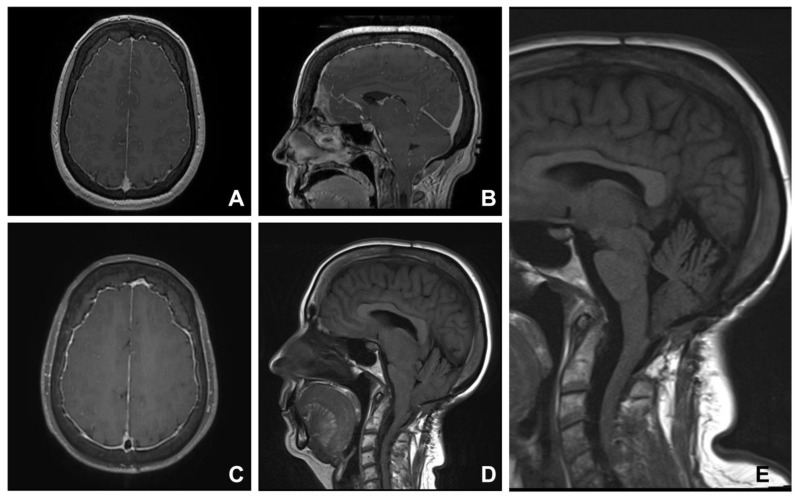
Chiari mimic: spontaneous intracranial hypotension. A 51-year-old woman was diagnosed with Chiari I malformation and syringomyelia and underwent posterior fossa decompression surgery at another institution. Worsening neurological symptoms led to further investigation for potential underlying spinal CSF leak. An MRI showed typical features of intracranial hypotension: diffuse pachymeningeal enhancement, pituitary gland enlargement, venous sinuses engorgement, and brain sagging appearance, an almost negligible pontomescencephalic angle, flattening of the pons, and cerebellar tonsillar descent (C,D). These features were present in the original pre-operative imaging (A,B). Surgical exploration at T8 confirmed a ventral spinal dural defect, and surgical repair led to an improvement in symptoms. In addition, post-operative imaging showed a restoration of pontomescencephalic angle, and interpeduncular and prepontine cisterns (E).
